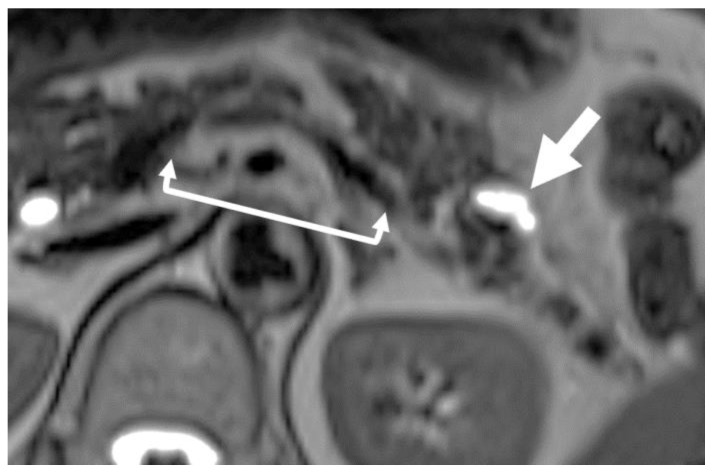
Focal pancreatic parenchymal atrophy (FPPA). T2 magnetic resonance imaging shows that the pancreatic parenchyma is lacking with an irregular shape and is replaced by fat (square arrow) near the cystic lesion (arrow).
